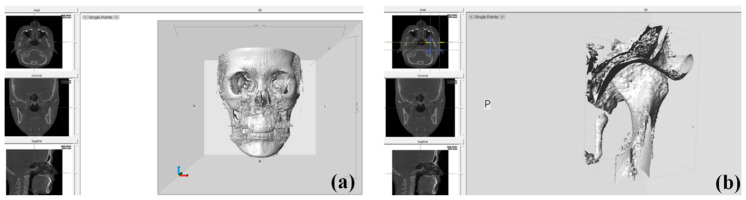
The view of Checkpoint (Stratovan): (a) imported CBCT data that were exported in DICOM format, (b) cropped volume isolating the left condyle-fossa unit.
